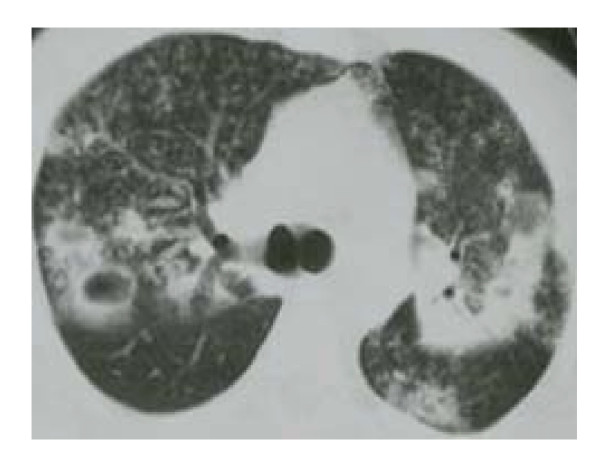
CT showing cavitary lesions in both lungs
